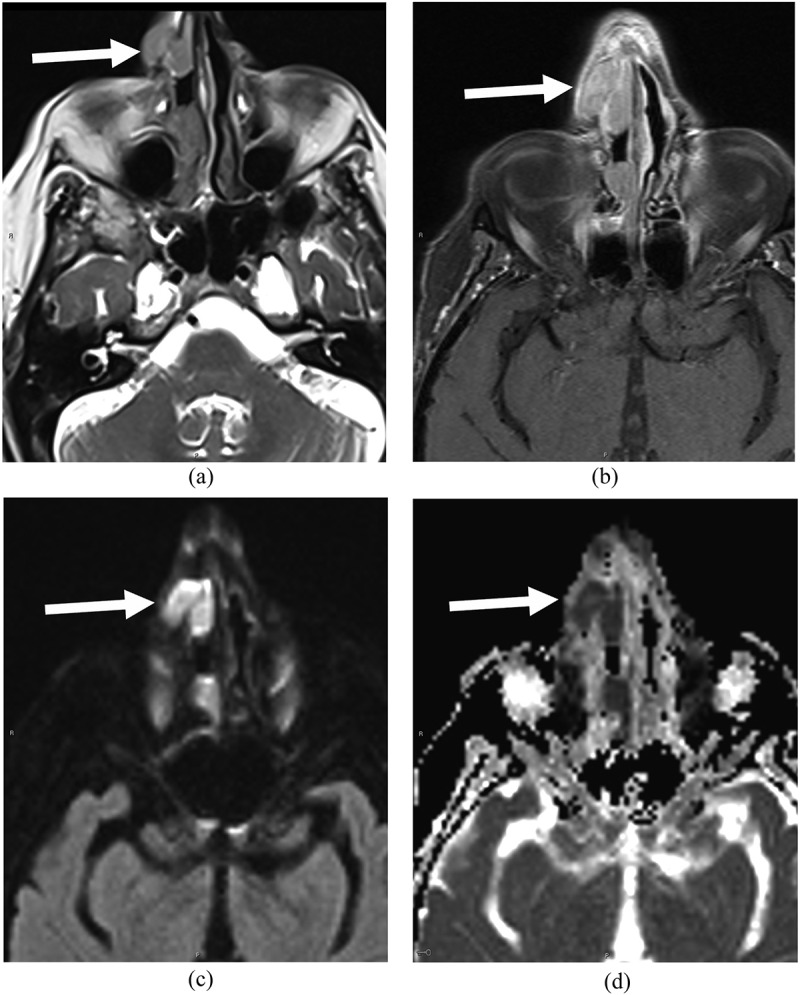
Axial MRI sinus showed soft tissue disease (arrows) in the right nasal cavity and right nasal alar region with low MRI T2 signal (a) and high T1 signal with contrast enhancement (b) and restricted diffusion on DWI/ADC (c, d).
